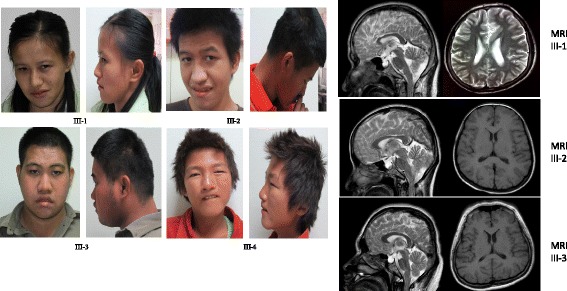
Clinical features of the patients. Left panel: III1-3: The craniofacial features of trisomy 11q23.3-q25 patients. Multiple facial dysmorphisms including microcephaly, brachycephaly, plagiocephaly and short philtrum are shown. III-4: The craniofacial features of the patient with Jacobsen syndrome. Her craniofacial features include facial asymmetry, low set ears, blepharophimosis, ptosis and epicanthal folds, broad nasal bridge, a thin upper lip. Right Panel: The brain MRI of 11q23.3-q25 trisomy patients. The brain MRI indicates the small pituitary gland and empty sella, and the bilateral parietal sulcus deepening was observed.
